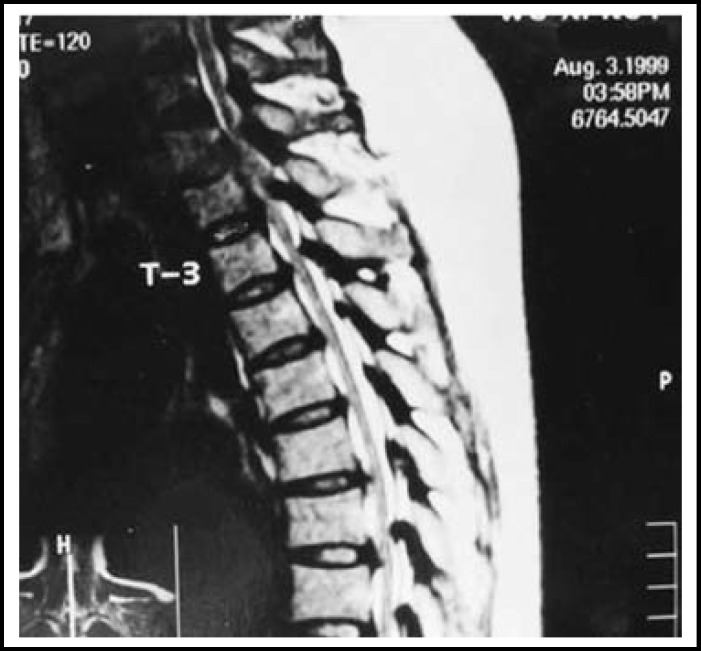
T2 weight MRI of thoracic spine showed multilevel cord compression by the bulging disc between C7 & T9 vertebrae
